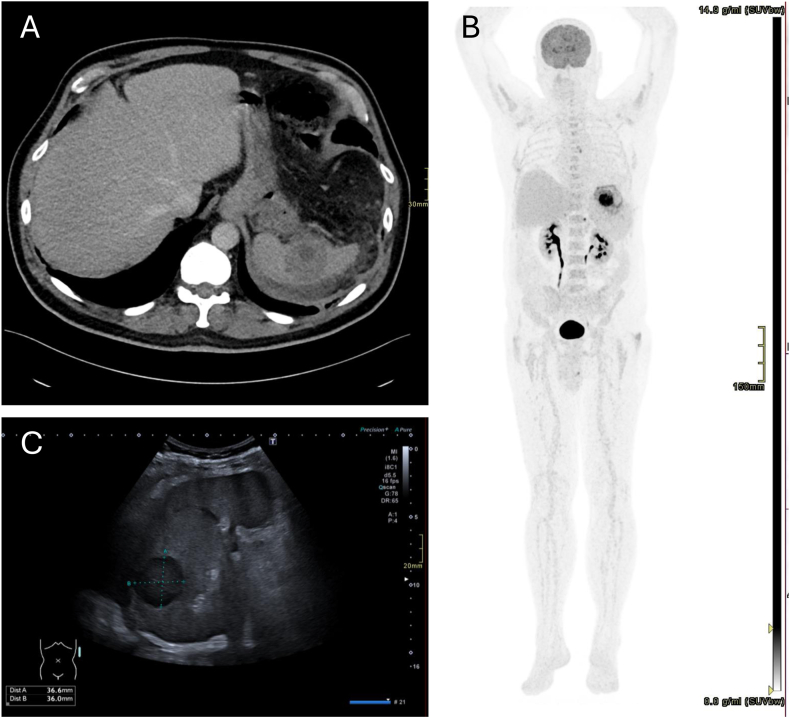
(A) Computed tomography revealing a large splenic abscess, soft tissue window, axial view. (B) Positron emission tomography ruled out metastatic infections, standardized uptake value, 0-15 g/mL. (C) Ultrasound of splenic abscess.
